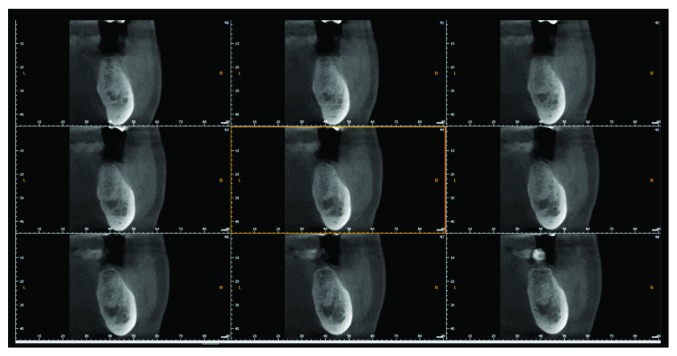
Para-axial cuts of a control CBCT before implant placement showing a complete bone regeneration of the cyst area.
